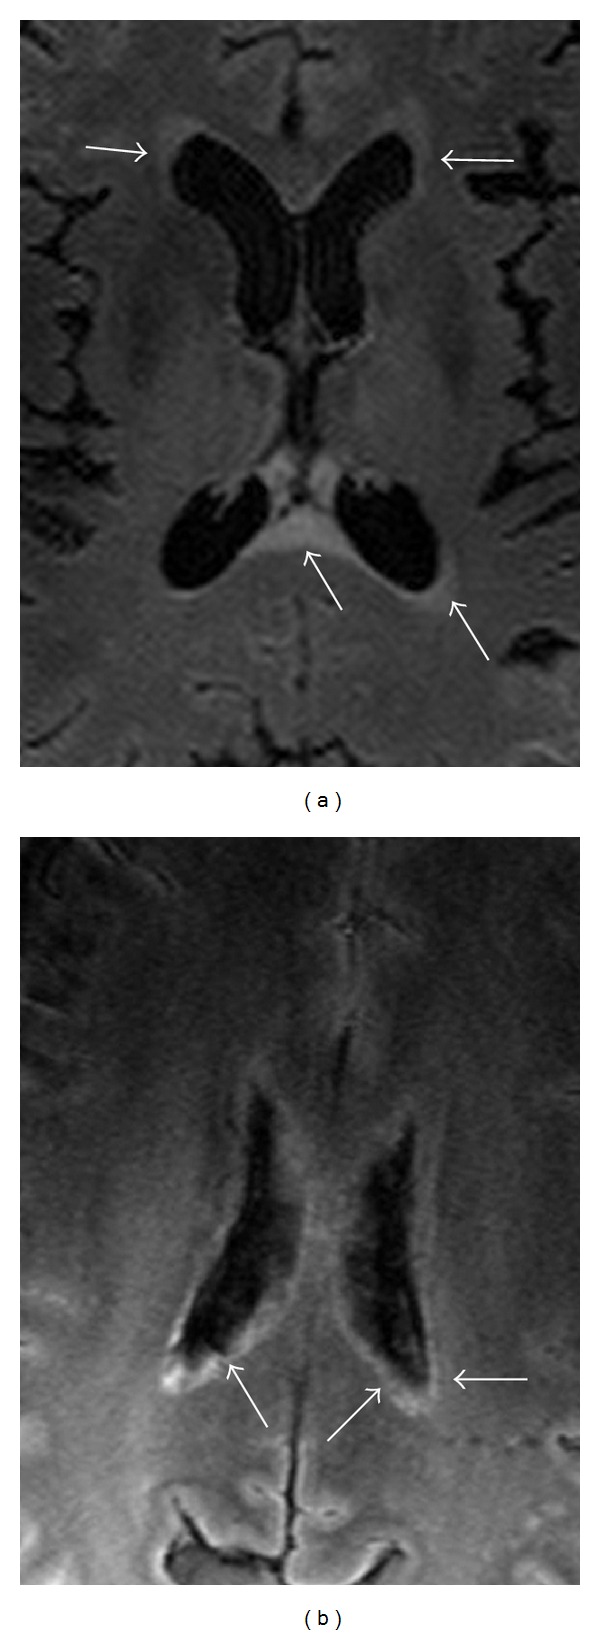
Examples of diffuse periependymal abnormalities. Abnormal and thickened signal intensities (arrows) along ependymal layers on FLAIR images in two patients ((a) and (b)).
